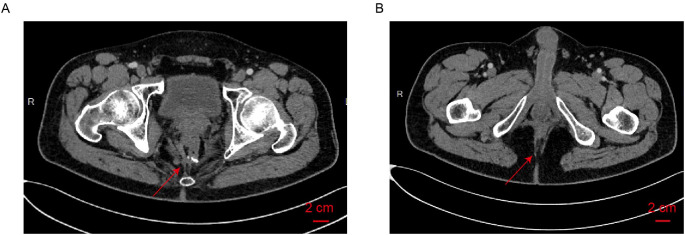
No evidence of tumor recurrence or metastasis was observed on the most recent CT examination (2025-09-24). (A): Axial CT of the rectal anastomosis. The arrow indicates the rectal anastomosis with no evidence of local recurrence. (B): Axial CT of the anus. The arrow indicates anus with no evidence of local recurrence.
